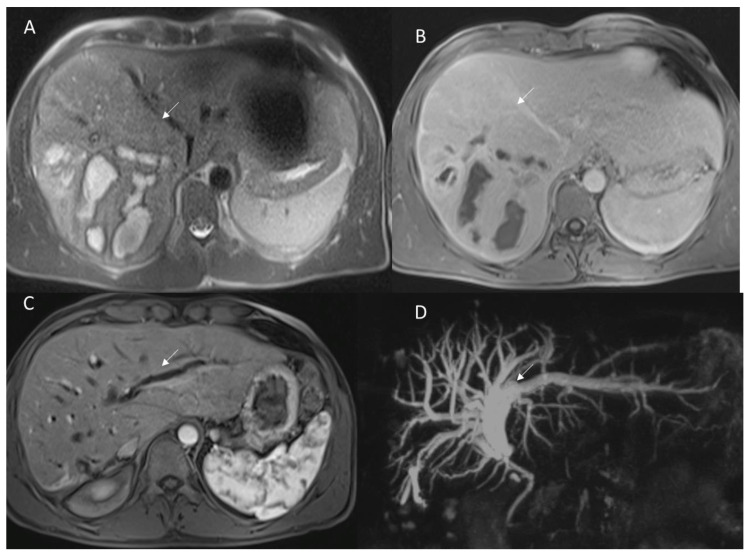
Patient with hepatosarcoma evaluated with MRI study ((A): T2-W sequence and (B): portal phase of contrast study). At MRI 6-month evaluation of arterial phase (C) and cholangiography (D) sequences show biliary strictures (arrow).
